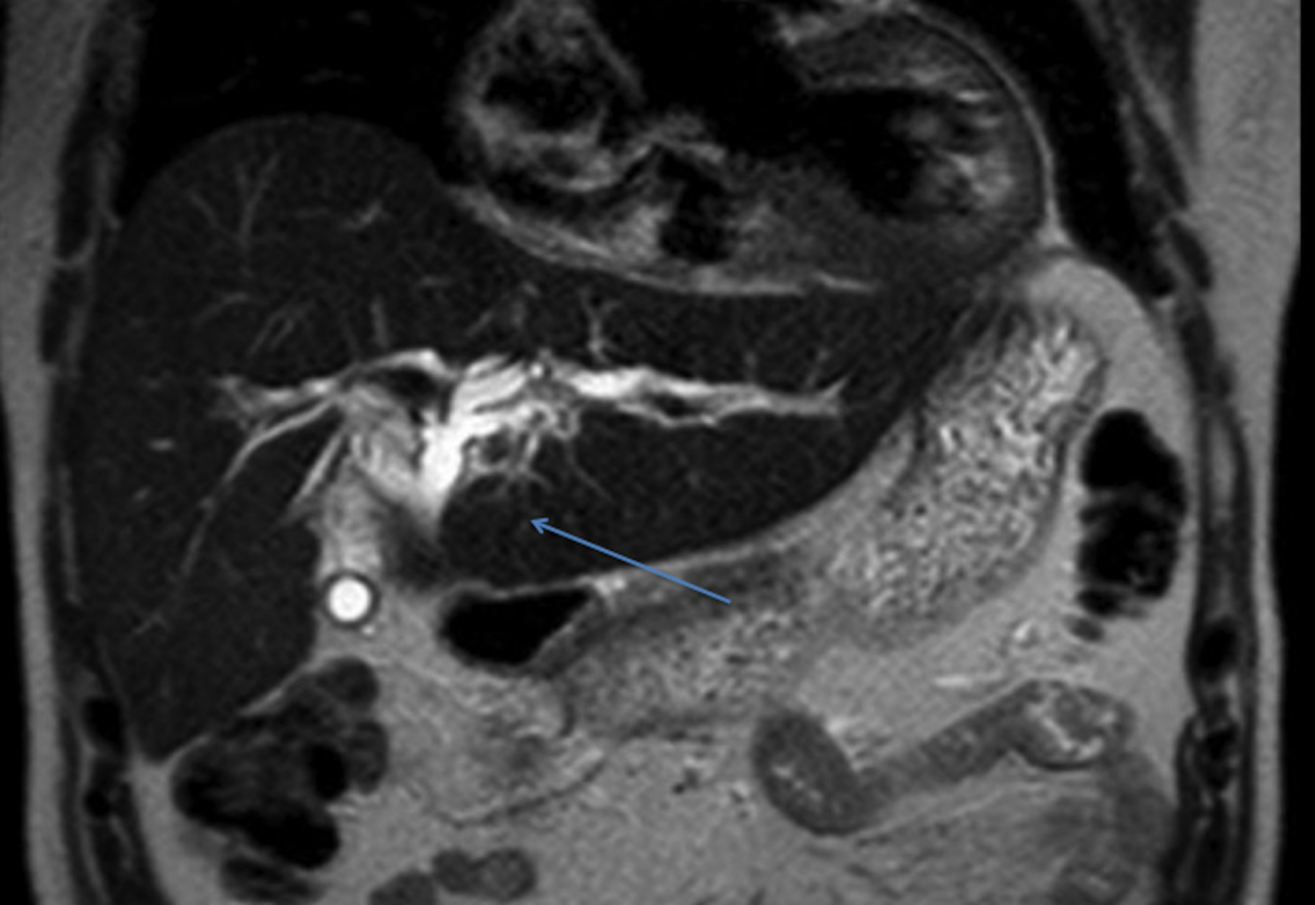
MRI of the abdomen demonstrating intrahepatic biliary dilation with no obvious mass MRI: Magnetic resonance imaging
